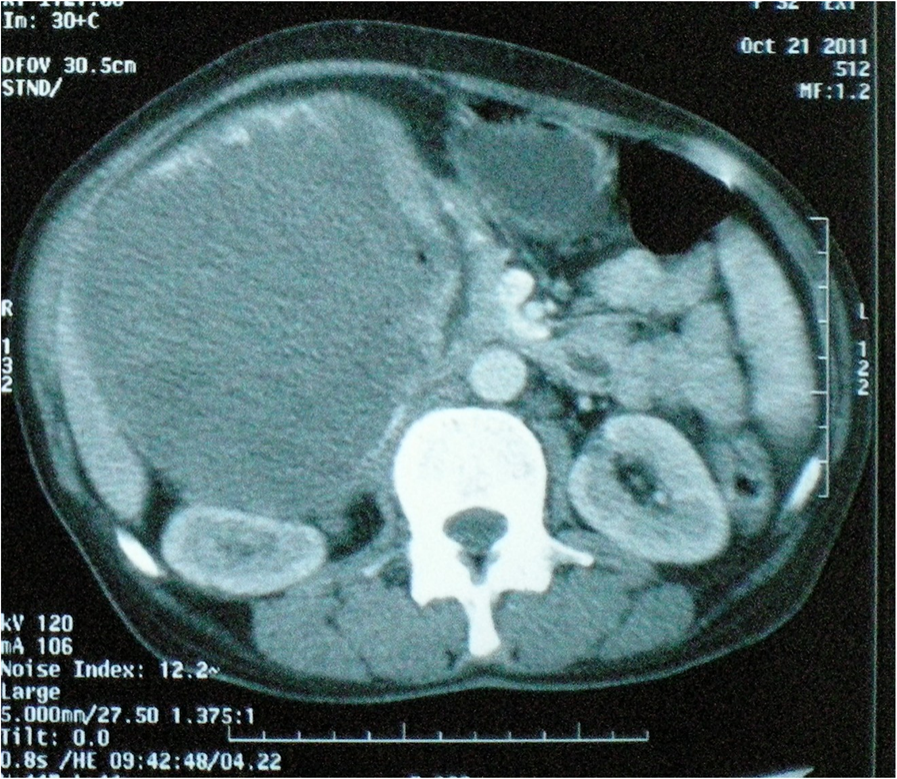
Abdominal computed tomography showing the large retropancreatic tumor.
